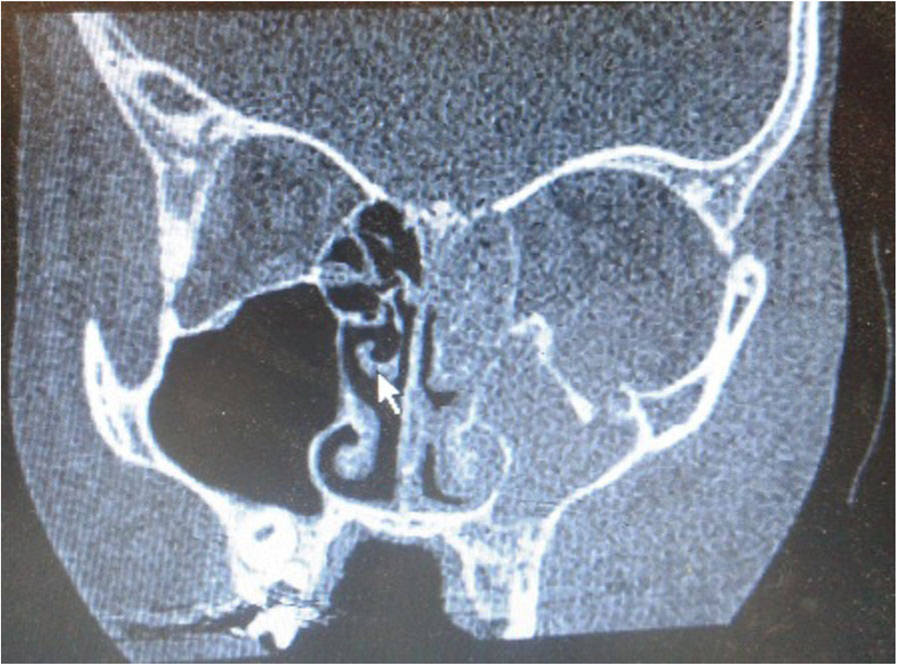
Inferior orbital cellulitis secondary to blow out fracture, Orbital CT scan coronal view revealed inferior wall fracture with total opacity of the left maxillary and ethmoid sinuses
